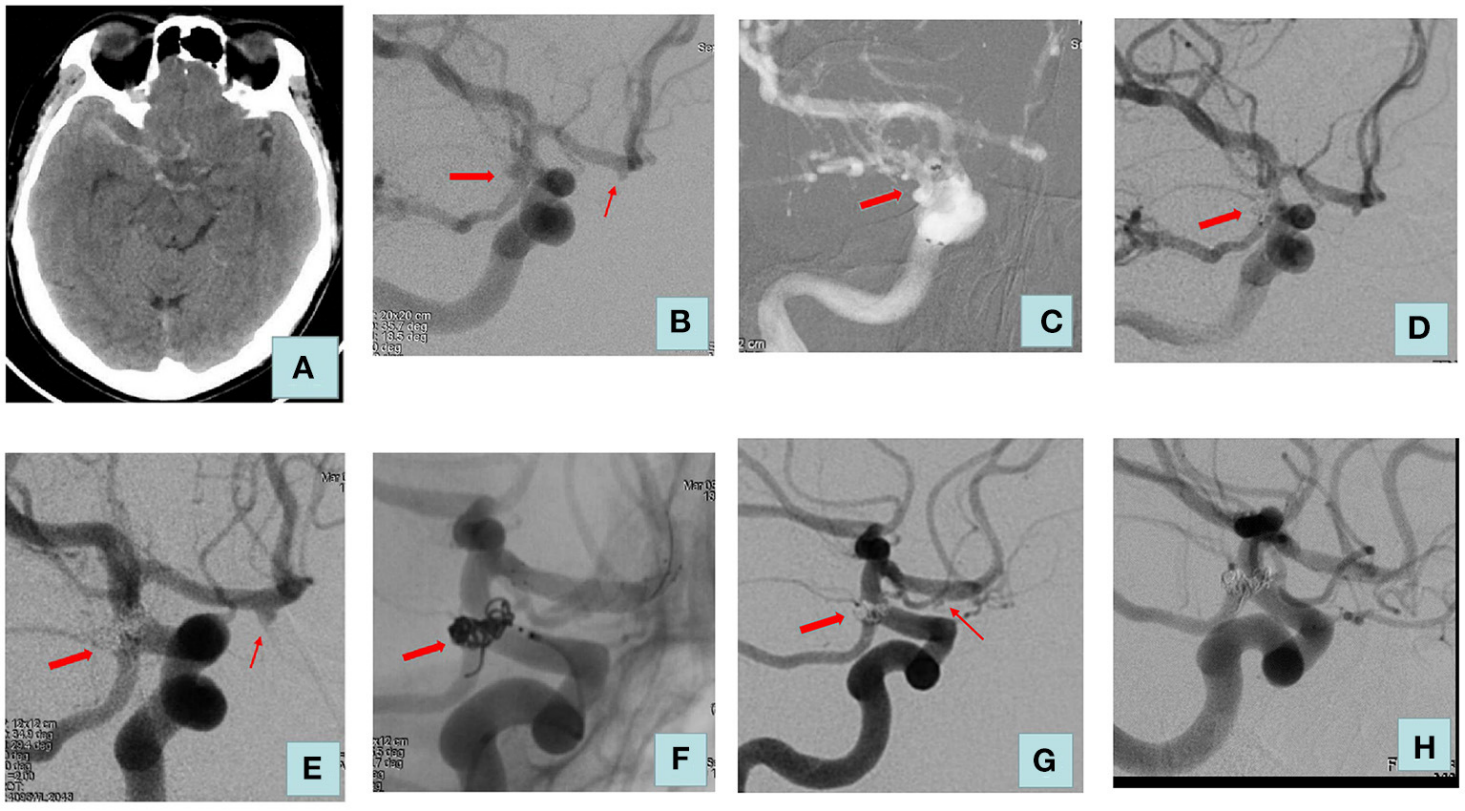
Adult patient presented with severe headache for 3 days. (A) Computed tomography of the head revealed a diffuse subarachnoid hemorrhage. (B) Cerebral angiography showed a right posterior communicating aneurysm (size: 4.2 * 3.8 mm) and an aneurysm of the right anterior cerebral artery (size: 2.2 * 1.8 mm). The posterior communicating aneurysm was considered to be a ruptured aneurysm. (C) Double microcatheter delivery to the aneurysm lumen. (D) We embolized the ruptured aneurysm using coils alone, and the Raymond–Roy classification immediately was complete embolization. (E) No recurrence of posterior communicating artery aneurysm at a 6-month postoperative review. (F) Treatment of unruptured aneurysm with one LVIS stent. (G) Raymond–Roy classification immediately was sac remnant (right anterior cerebral artery). (H) Review 2 years after surgery: posterior communicating artery aneurysm and anterior cerebral artery aneurysm were complete embolization. The thick arrow indicates a ruptured aneurysm; the thin arrow indicates an unruptured aneurysm.
